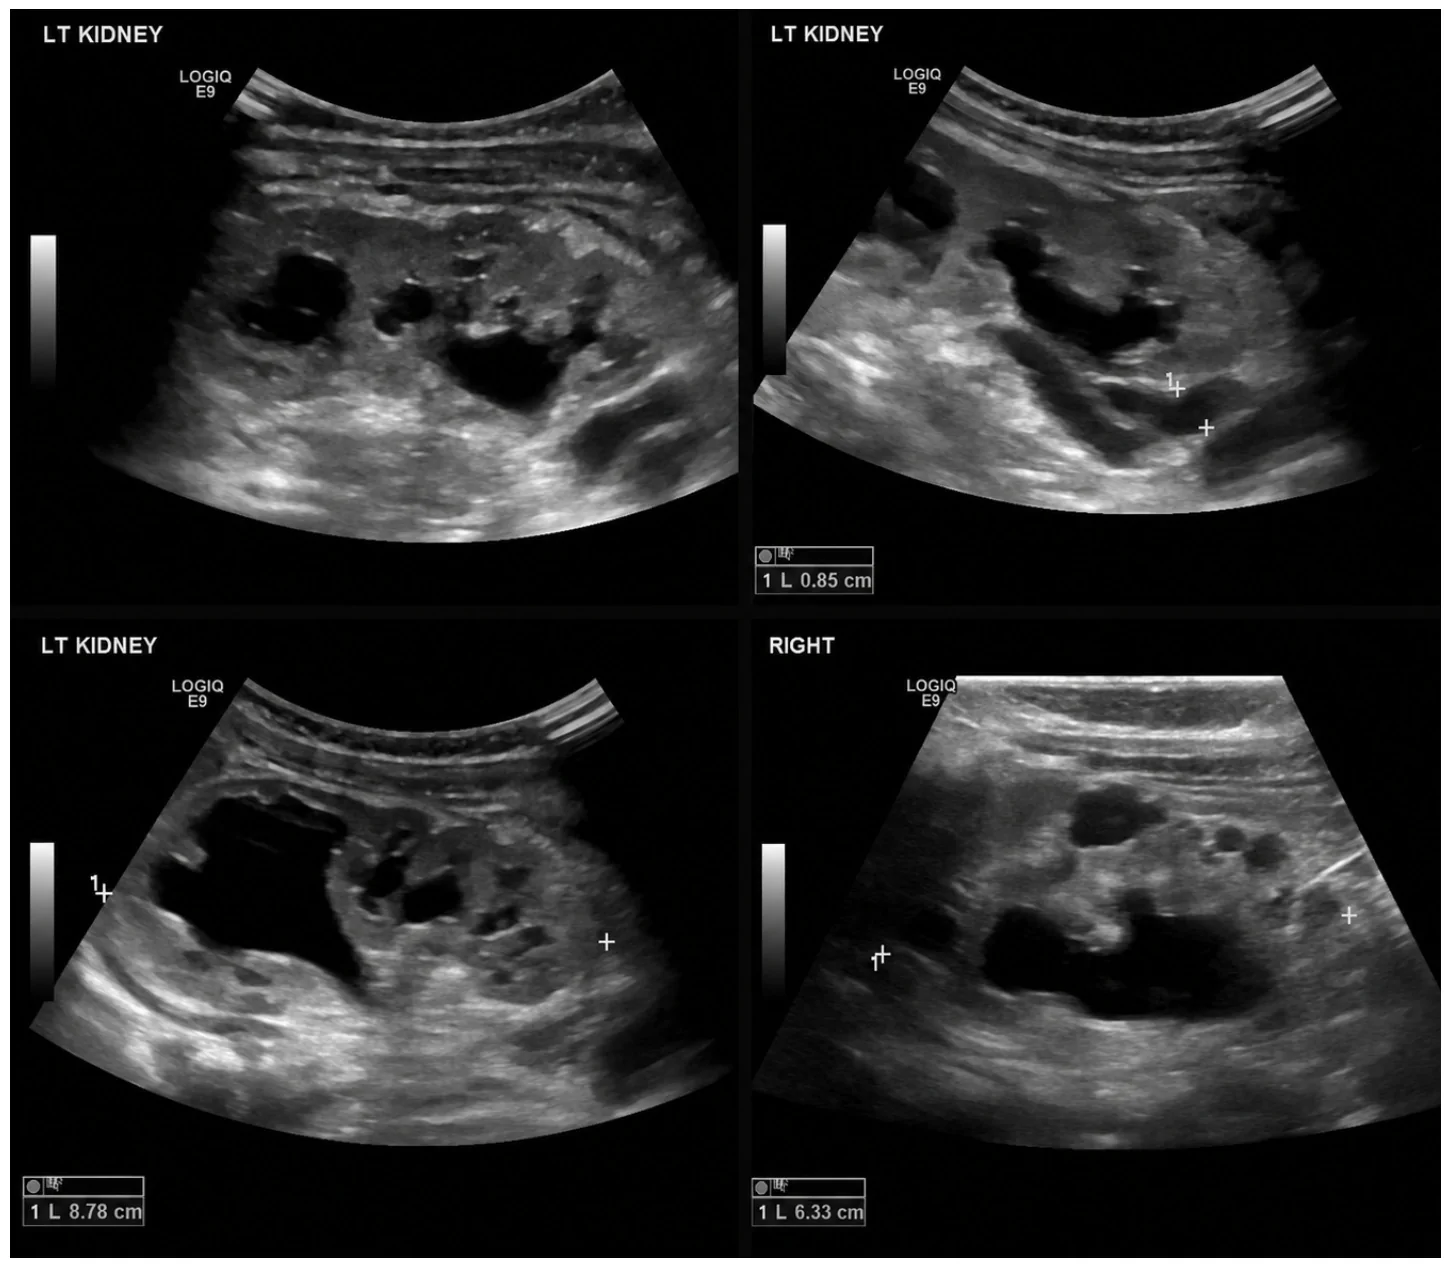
Post-op renalultrasound demonstrating worsening hydronephrosis of both the upper- and lower-pole moieties of the left duplex collecting system, with persistent ureteral dilatation. The contralateral right MCDK is also shown.
